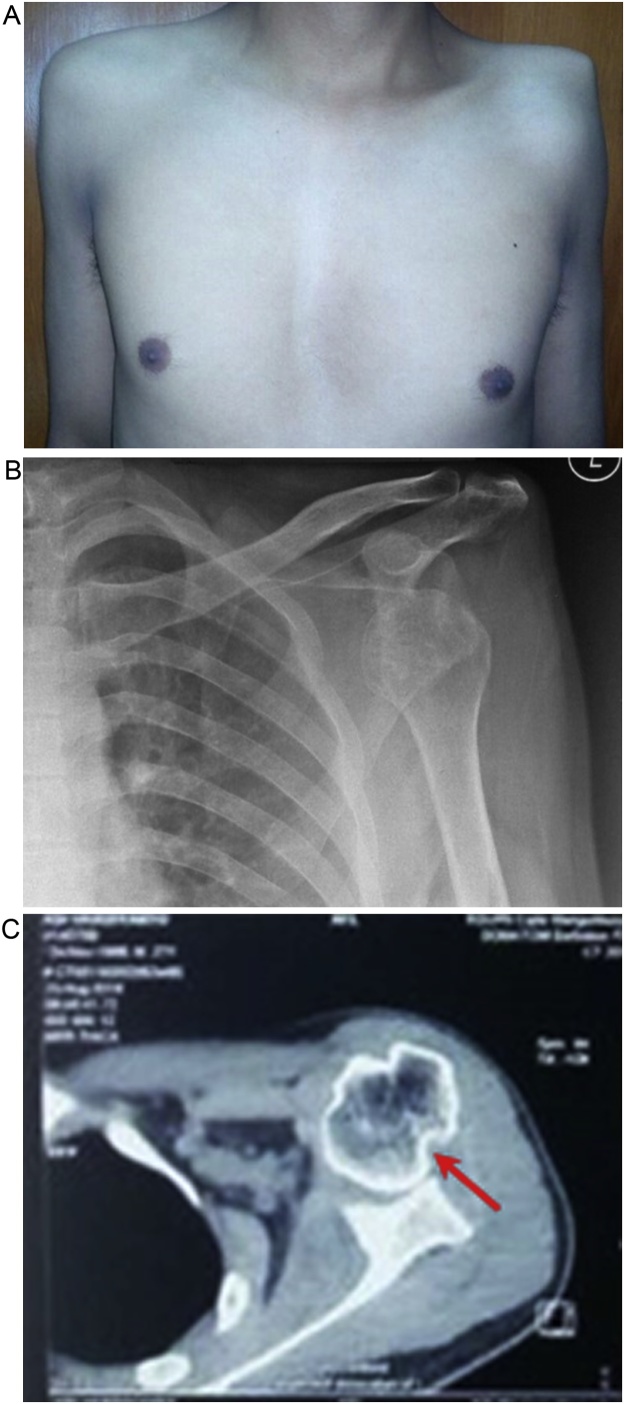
(a) Clinical picture of bilateral shoulder showing a squaring left shoulder with muscle atrophy. (b) X-ray antero-posterior view of the left shoulder showing anterior dislocation of glenohumeral joint. (c) CT scan showed a Hill-Sachs lesion on the humeral head (pointed by arrow).
